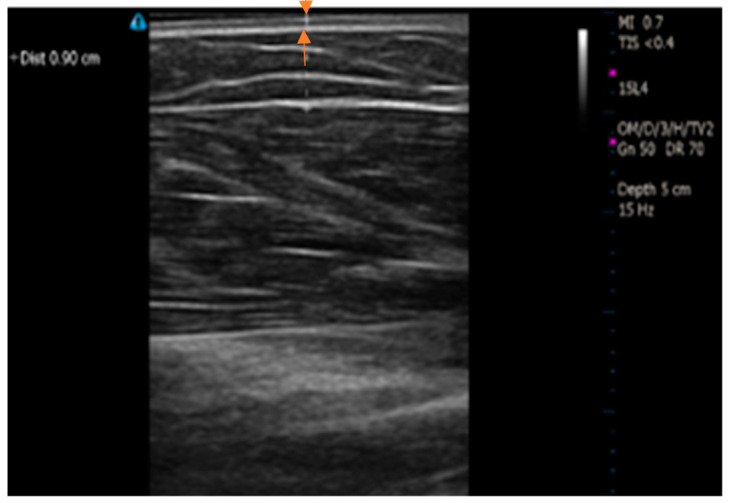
Ultrasound image of skin thickness of the human subject.
